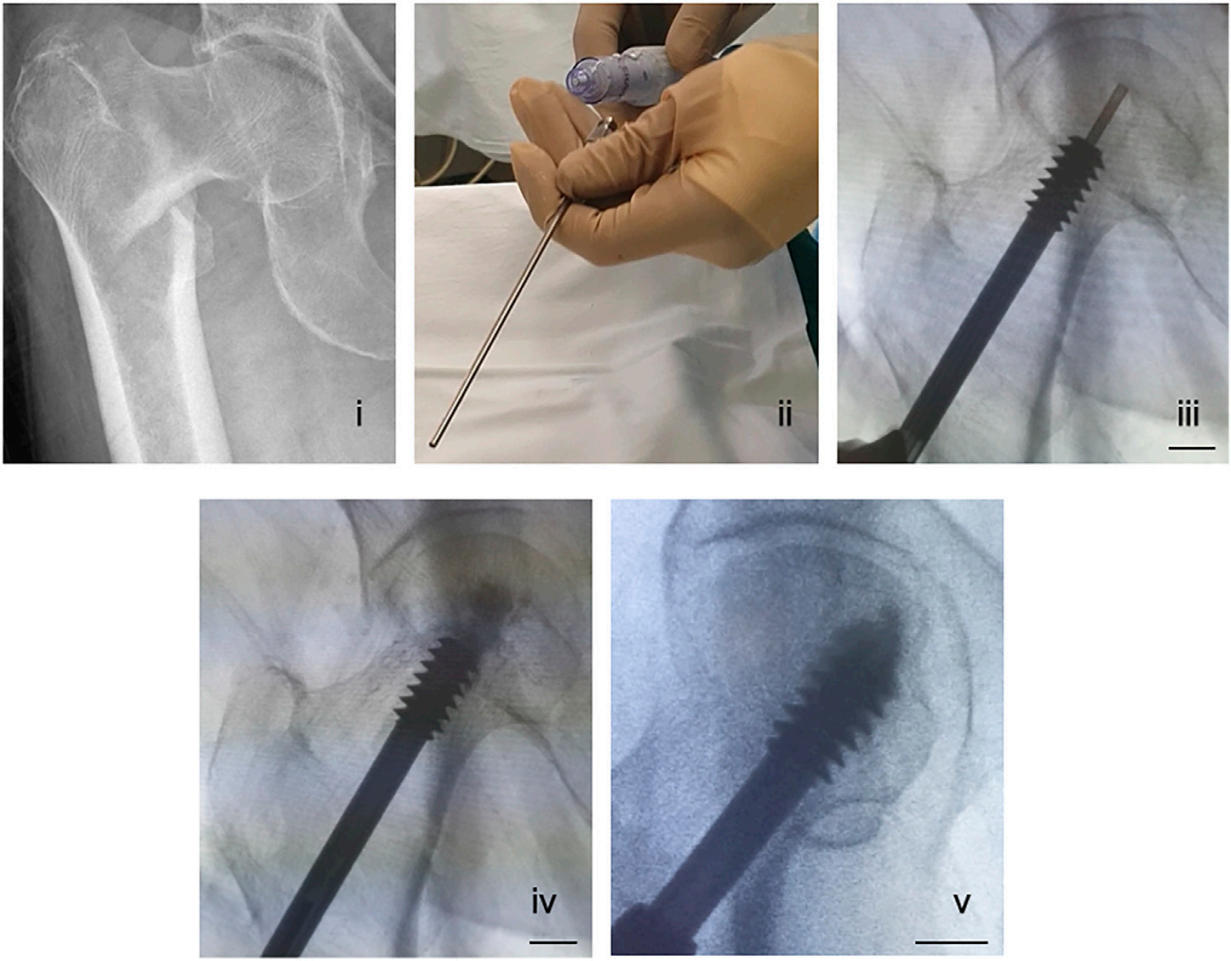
A surgical method of delivering a CaS/HA biomaterial through a cannulated lag-screw in a patient undergoing treatment for pertrochanteric fracture. (i) X-ray showing a pertrochanteric fracture. (ii) A photograph of the injection cannula being connected to a regular injection syringe containing the CaS/HA biomaterial. (iii) Placement of the titanium cannula at the most distal end of the pre-drilled canal via the cannulated lag-screw visualized radiographically. (iv) Radiographic image of the CaS/HA biomaterial being injected into the pre-drilled canal in-front of the lag-screw. (v) Radiograph of the final position of the lag-screw with the biomaterial around. Scale bar in panels iii-v is ∼1.3 cm.
